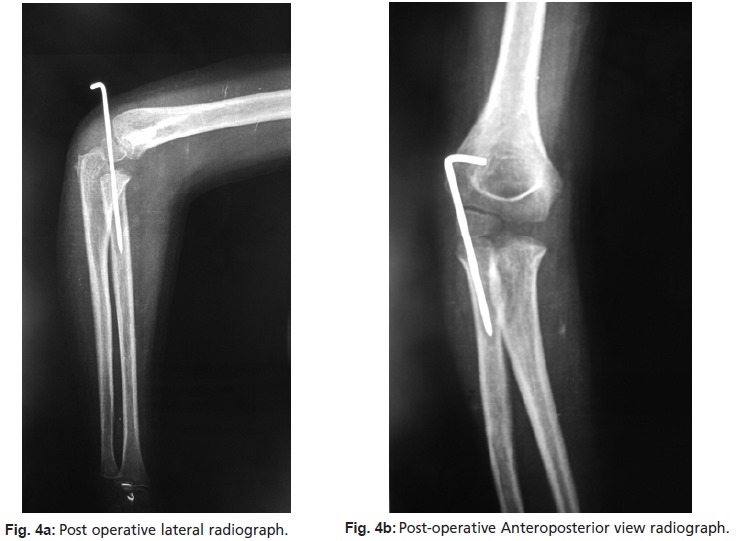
4(a)Post operative lateral radiograph.
